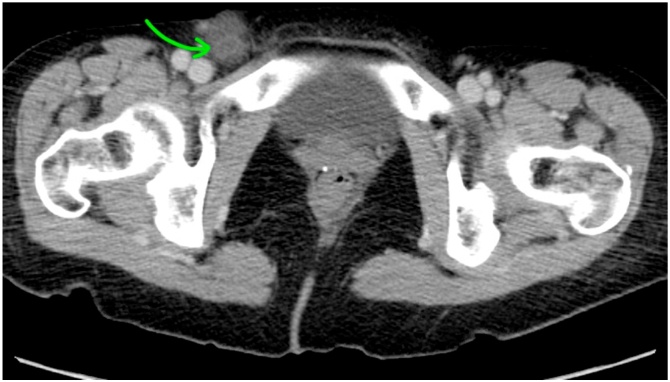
Computed tomography scan demonstrating right-sided inguinal hernia—in this case, a dilated appendiceal tip within the femoral canal (De Garengeot hernia).
